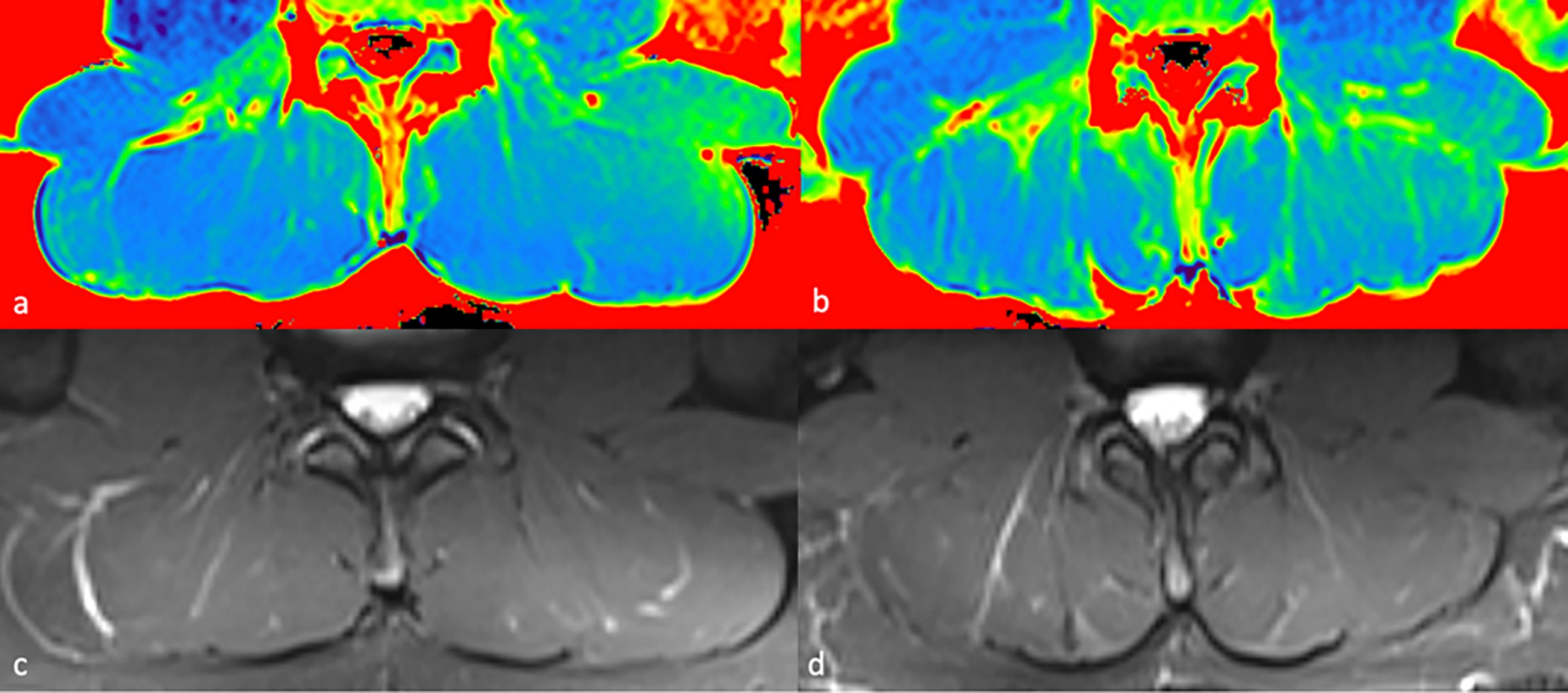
Color-coded ADC maps with corresponding fat-suppressed T2-weighted images. Exemplary color-coded ADC maps and T2-weighted inversion-recovery images of the lumbar spine of a 14-year old male scull rower (a,c) and a 15-year old male sweep rower (b,d). ADC values were significantly higher in sweep rowers. No pathological findings were evident on conventional images. ADC Apparent Diffusion Coefficient.
